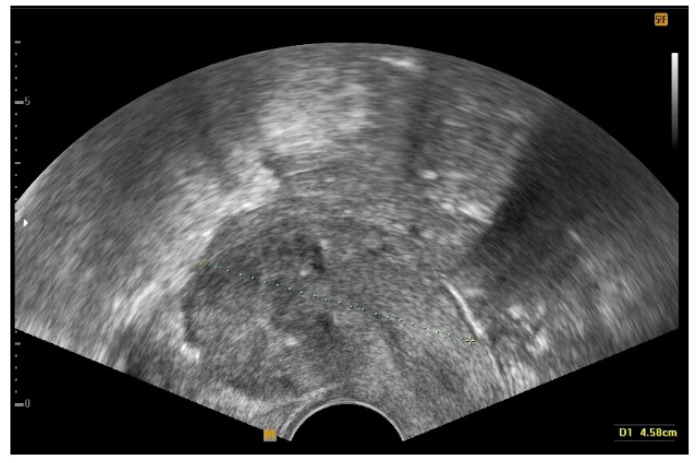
Transvaginal ultrasonography of the uterus during follow-up after the conservative surgery. The image shows a complete eradication of diffuse leiomyomatosis.
